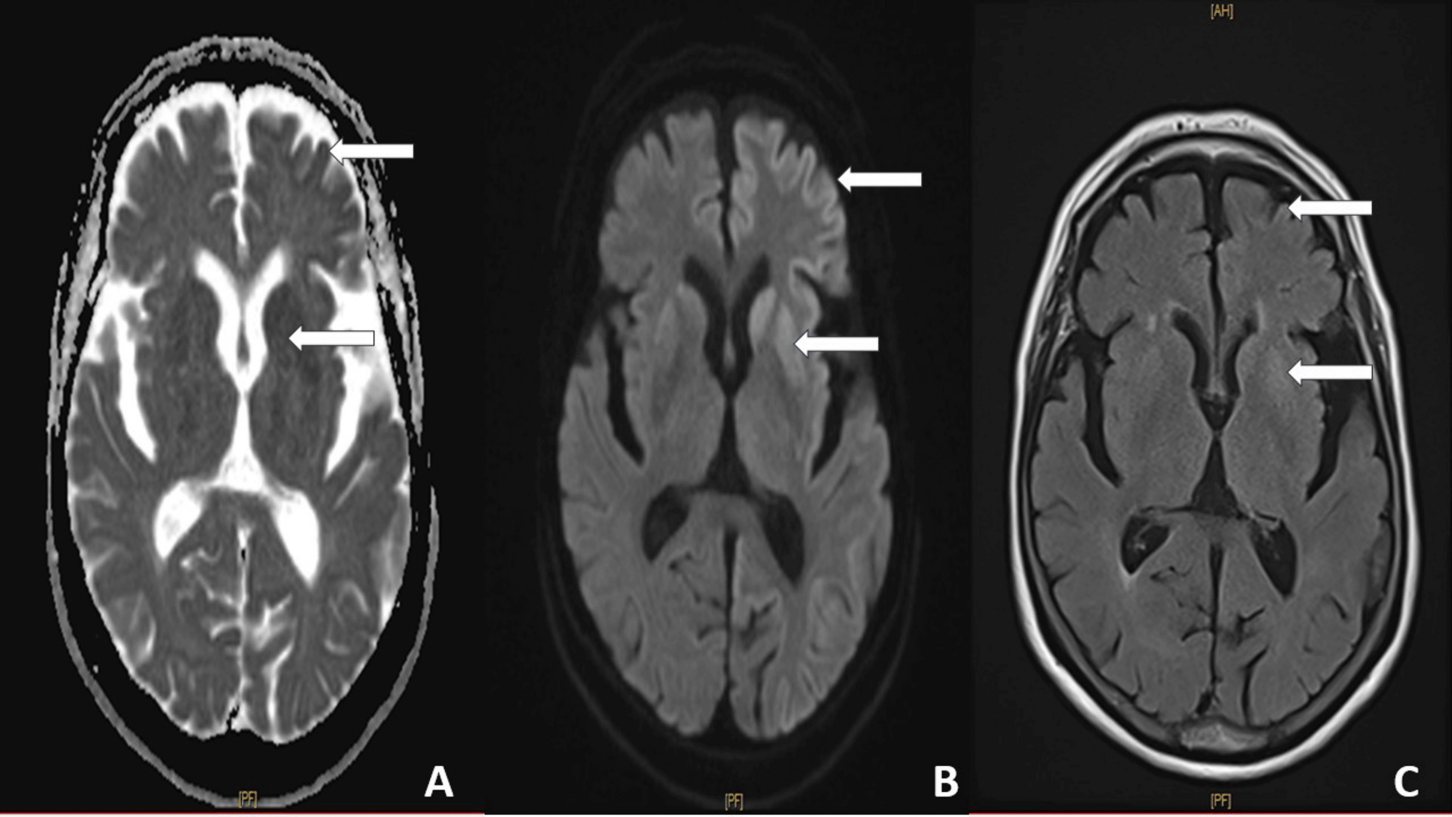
Brain MRI showing characteristic abnormalities in Creutzfeldt-Jakob disease. (A) Apparent diffusion coefficient (ADC) map demonstrating cortical and bilateral striatal hypointensity involving the caudate nuclei and putamina, consistent with true restricted diffusion (arrows). (B) Diffusion-weighted imaging (DWI) showing cortical ribboning and bilateral basal ganglia hyperintensity (arrows). (C) Fluid-attenuated inversion recovery (FLAIR) sequence revealing concordant cortical and striatal hyperintensity without mass effect (arrows). These diffusion abnormalities are considered highly sensitive neuroimaging markers of sporadic Creutzfeldt-Jakob disease and may appear early in the disease course.
